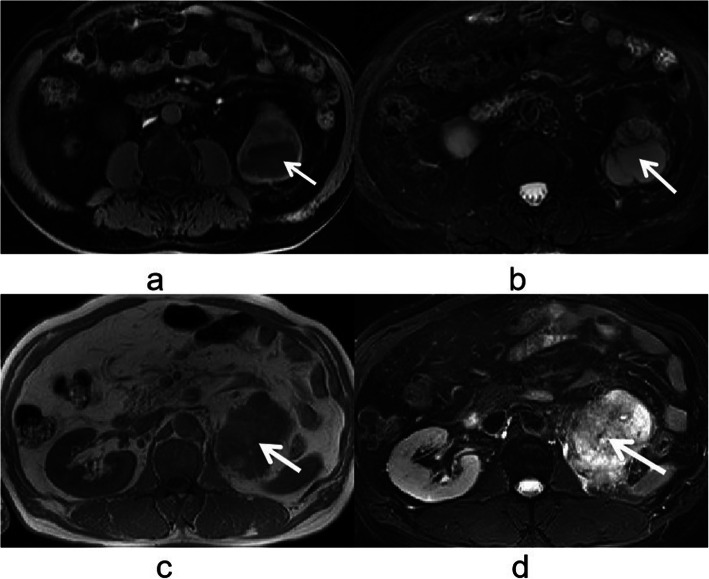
a-d. CRCC and CCDC of MR imaging. a CRCC appeared hypointense on T1-weighted images. b CRCC appeared hyperintense on T2-weighted images. c CCDC appeared hypointense on T1-weighted images. d CCDC appeared hypointense on T2-weighted images
